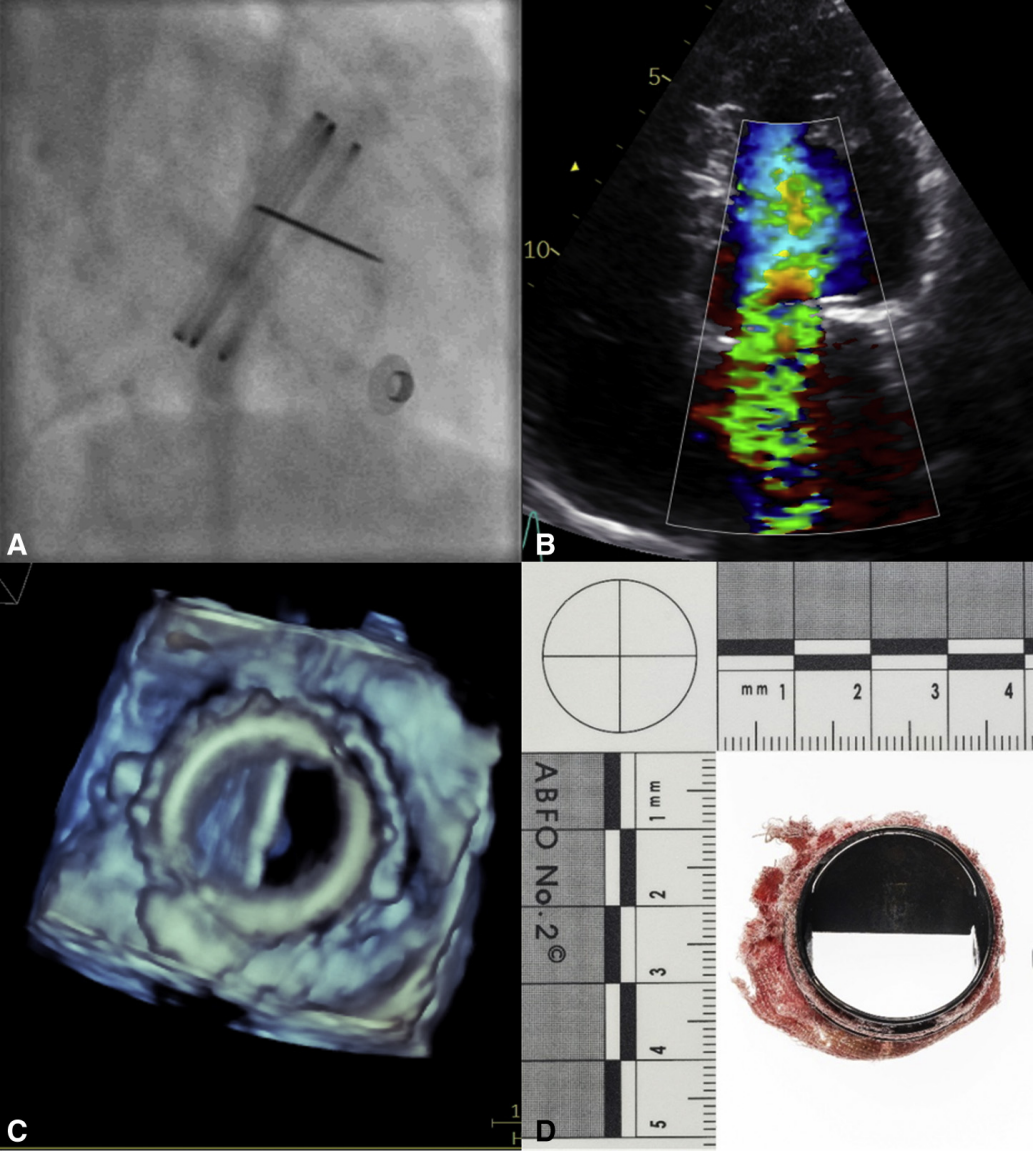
Fluoroscopy (A), transthoracic echocardiography (B), and transesophageal echocardiography with 3D reconstruction (C) of a patient in cardiogenic shock showing the mitral prosthesis with 1 missing leaflet and significant regurgitation. D, After emergency mitral valve replacement, the explanted prosthesis with 1 leaflet clearly missing.
